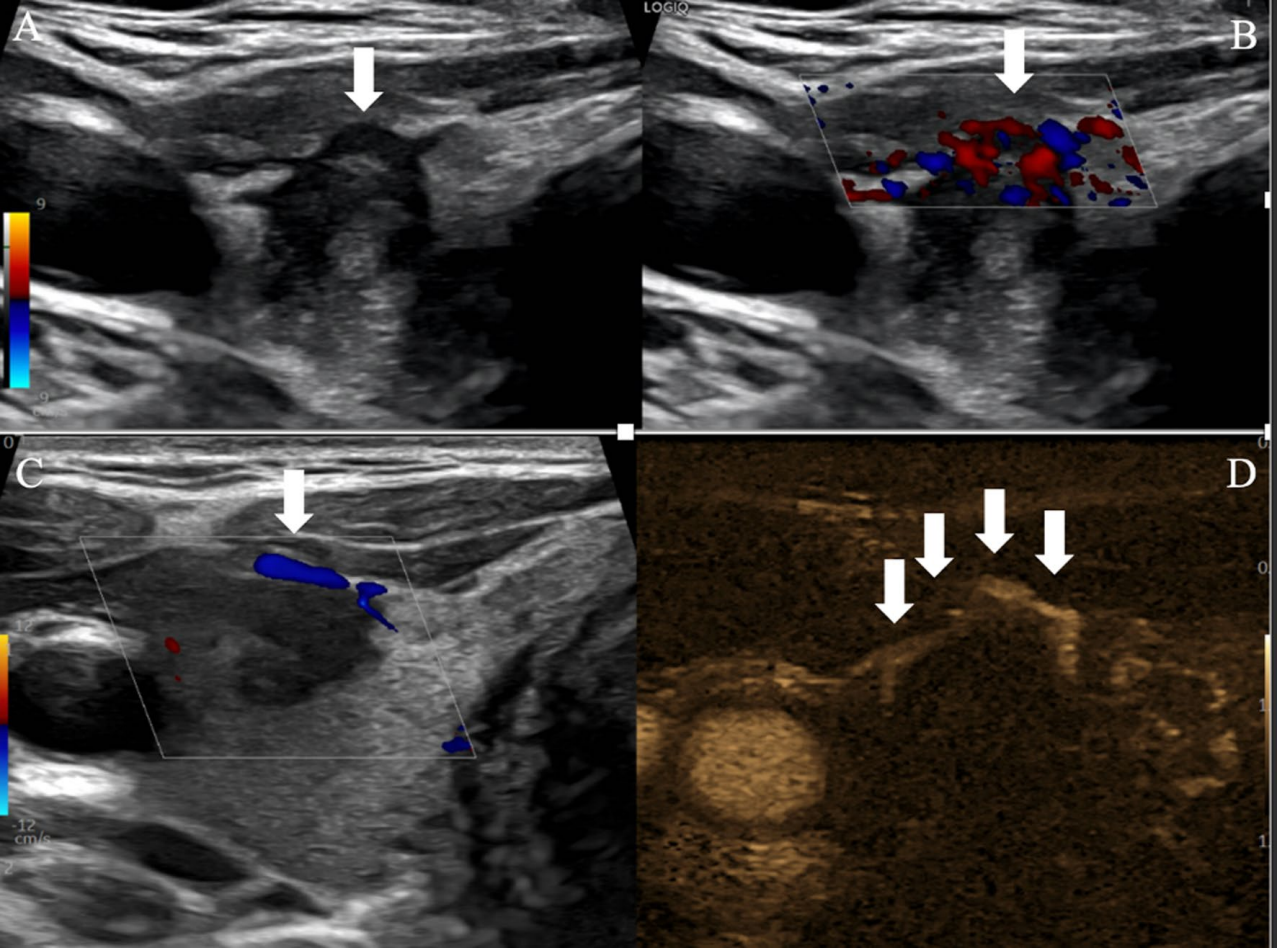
US assessment of PTC extra thyroid extension (ETE). Axial US scan, grey scale (A), colour Doppler (B, C), MicroVascular Imaging MVI (D) of right lobe PTC. Contour bulging is shown (arrow, A), neovasculature (arrow) is seen running on the capsula and increases sensitivity of ETE diagnosis (B). In case of anterior abutment without disruption (C, D), Doppler US discloses vessel (arrow) running along the, while MVI depicts more subtle capsular vessels (arrows)
